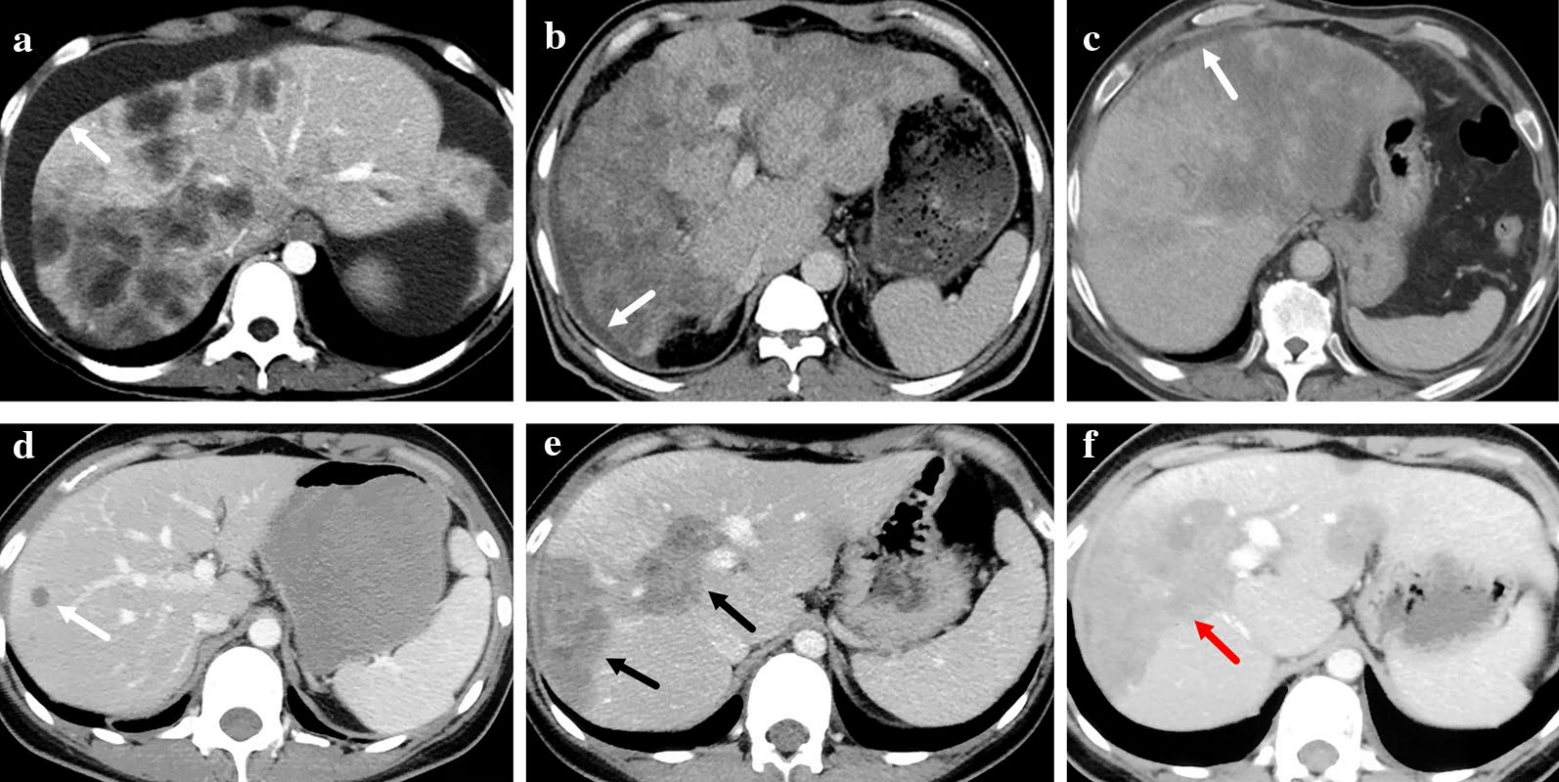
a–c Perihepatic effusion of three HEH patients on CT scan (marked with white arrows). d–f The changes of CT appearances of a 28-year-old male HEH patient. During the period of 6 years follow-up, the patient received no treatment. d At the time of diagnosis, nodular lesion could be found (marked with a white arrow). e The CT scan at 4 years after the diagnosis showed locally coalescent lesions (marked with black arrows). f The CT scan at 6 years after the diagnosis showed diffusely coalescent lesion (marked with a red arrow)
